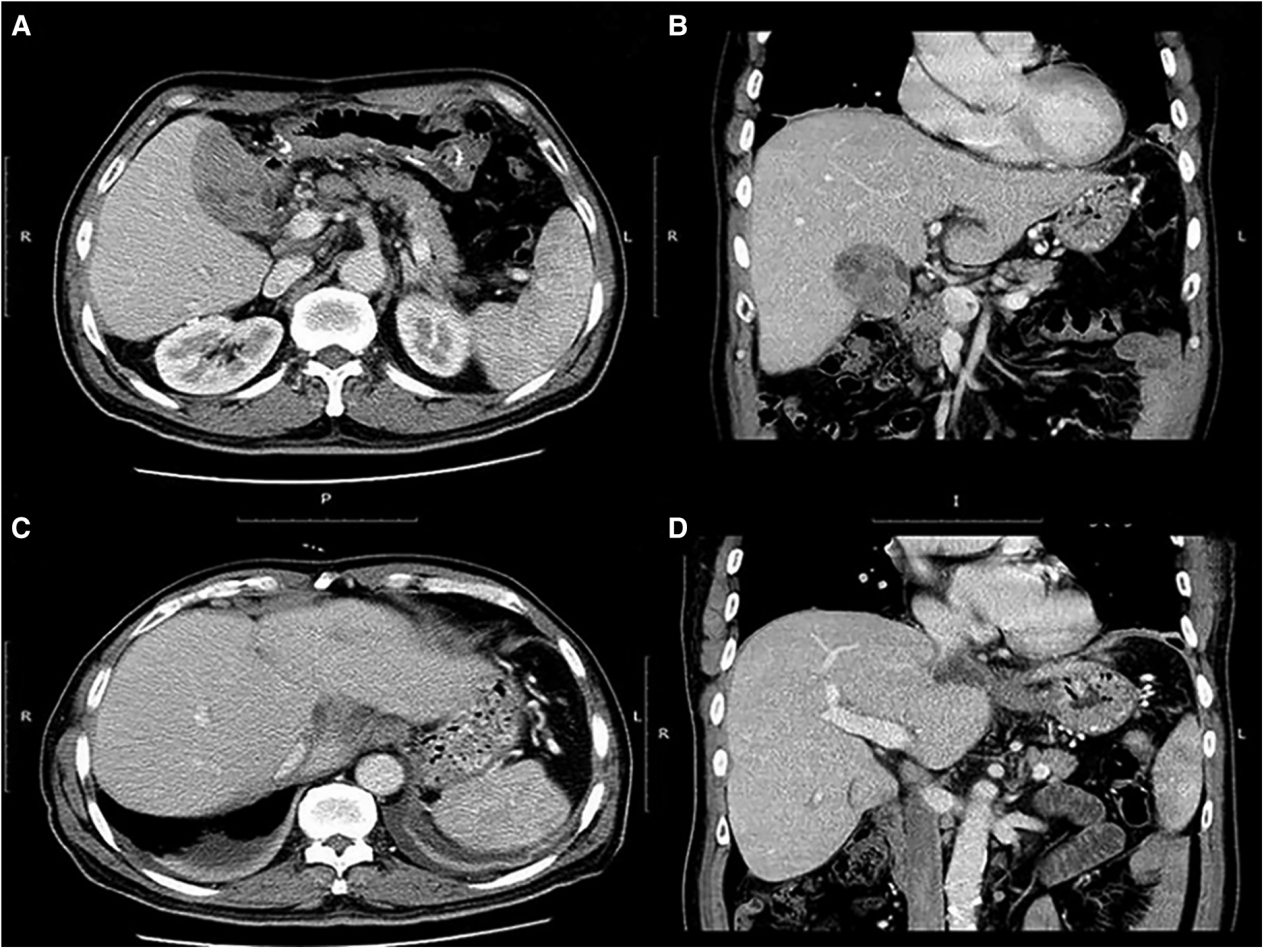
(A) computed tomography (CT) depicted a gallbladder tumor with dimensions of 8 × 4 cm. (B) Coronal image shows a clear demarcation between the tumor and the adjacent liver parenchyma. (C) No perceivable intrahepatic foci were found. (D) The right portal vein is tumor thrombus free.
